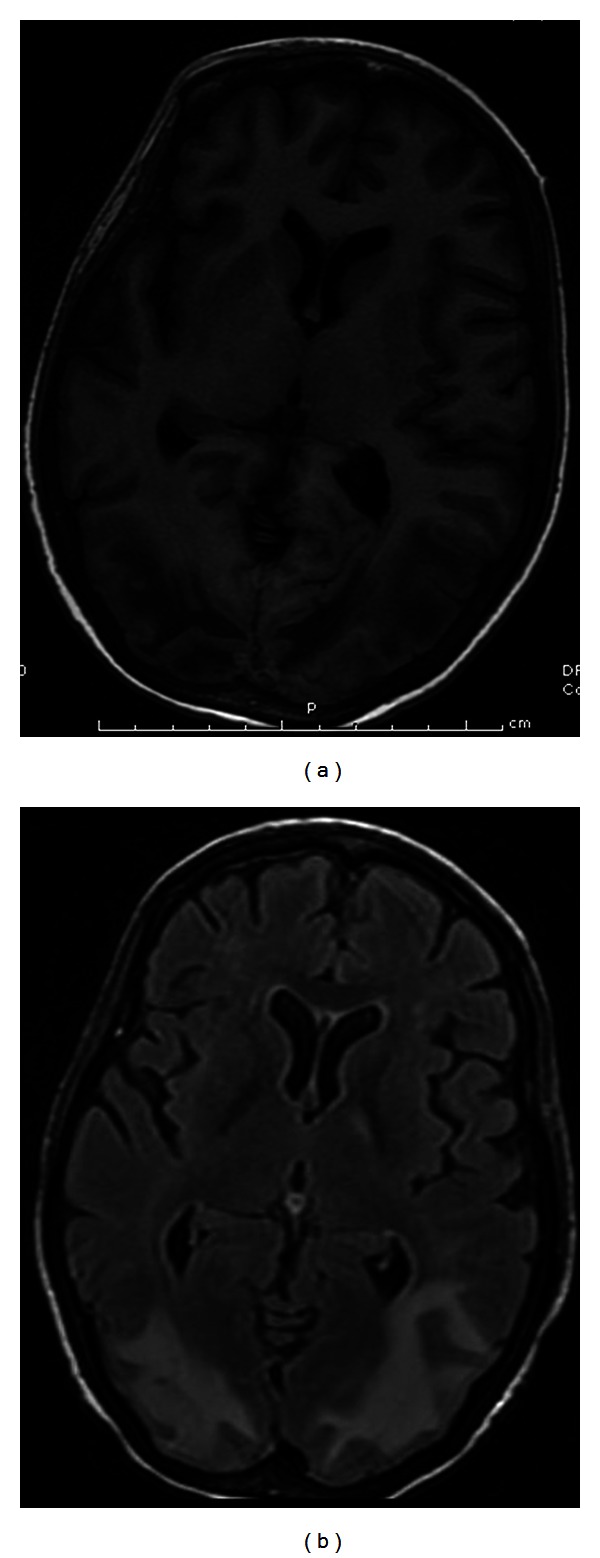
(a) Axial T1-weighted MRI image showing hypointensity in the occipital and parietal regions bilaterally. (b) Axial FLAIR with gadolinium MRI image showing lack of enhancement.
